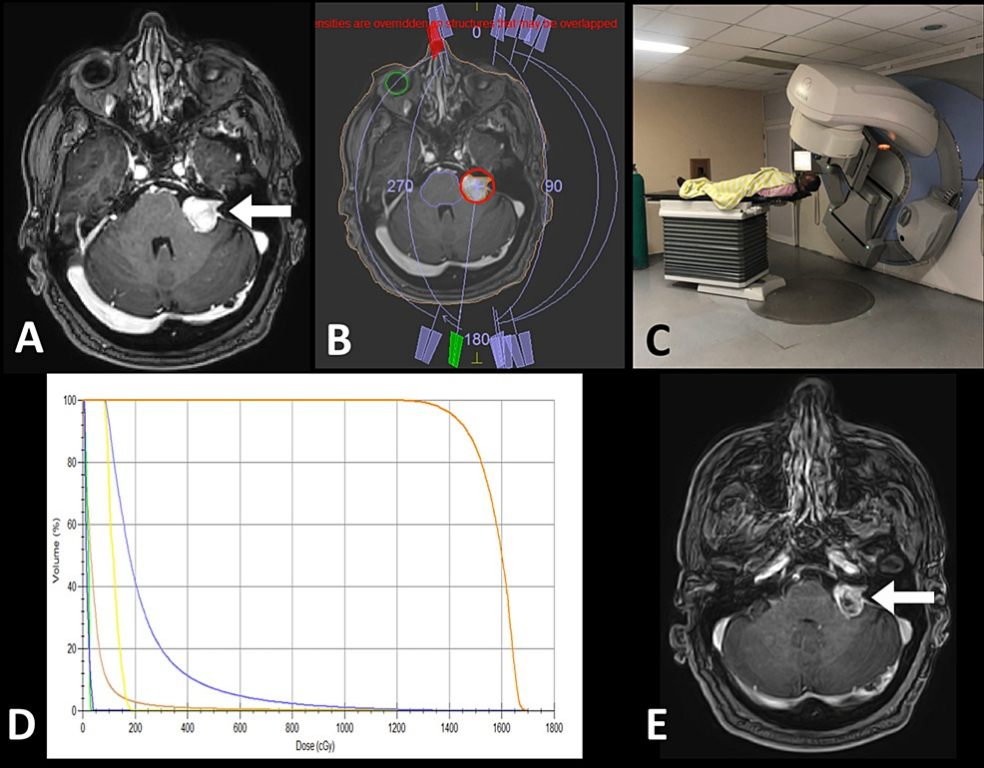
Patient with vestibular schwannoma. A: MRI planning. Arrow: Lesion at the left cerebellopontine angle. B: The planning CT image is observed with the treatment arches. C: Positioning of the patient in the linear accelerator (LINAC). D: Dose/volume histogram. E: MRI C+ at six months of treatment where partial regression and necrotic center are observed (arrow).
